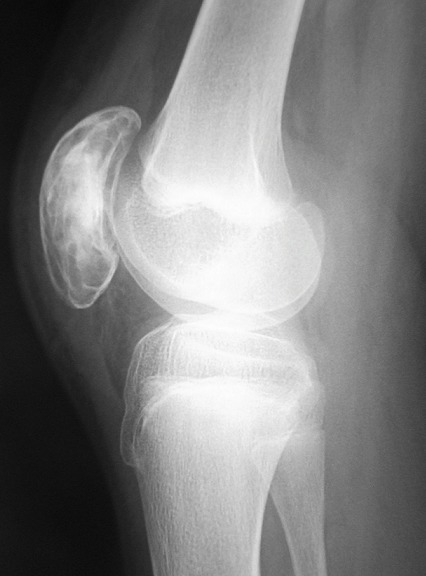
Radiography of the right knee showing the iliac graft packing of the patella
